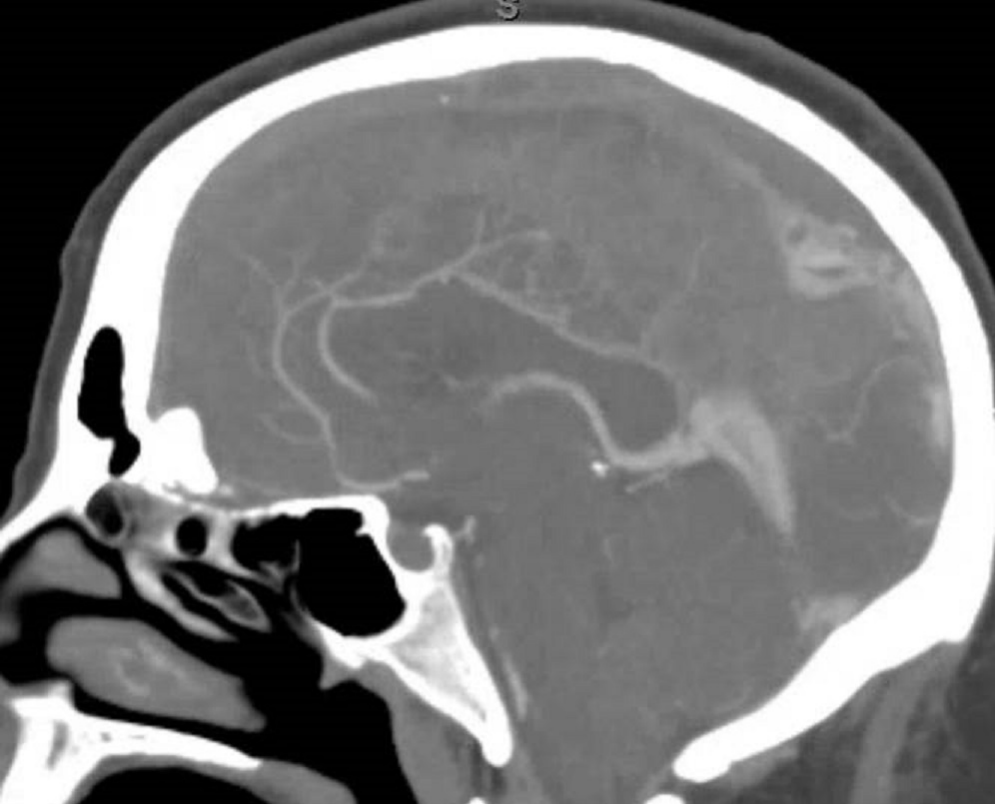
CT Angiogram at Presentation CT angiogram obtained at presentation in the sagittal view reveals no flow through sagittal sinus and flow through internal cerebral veins.
